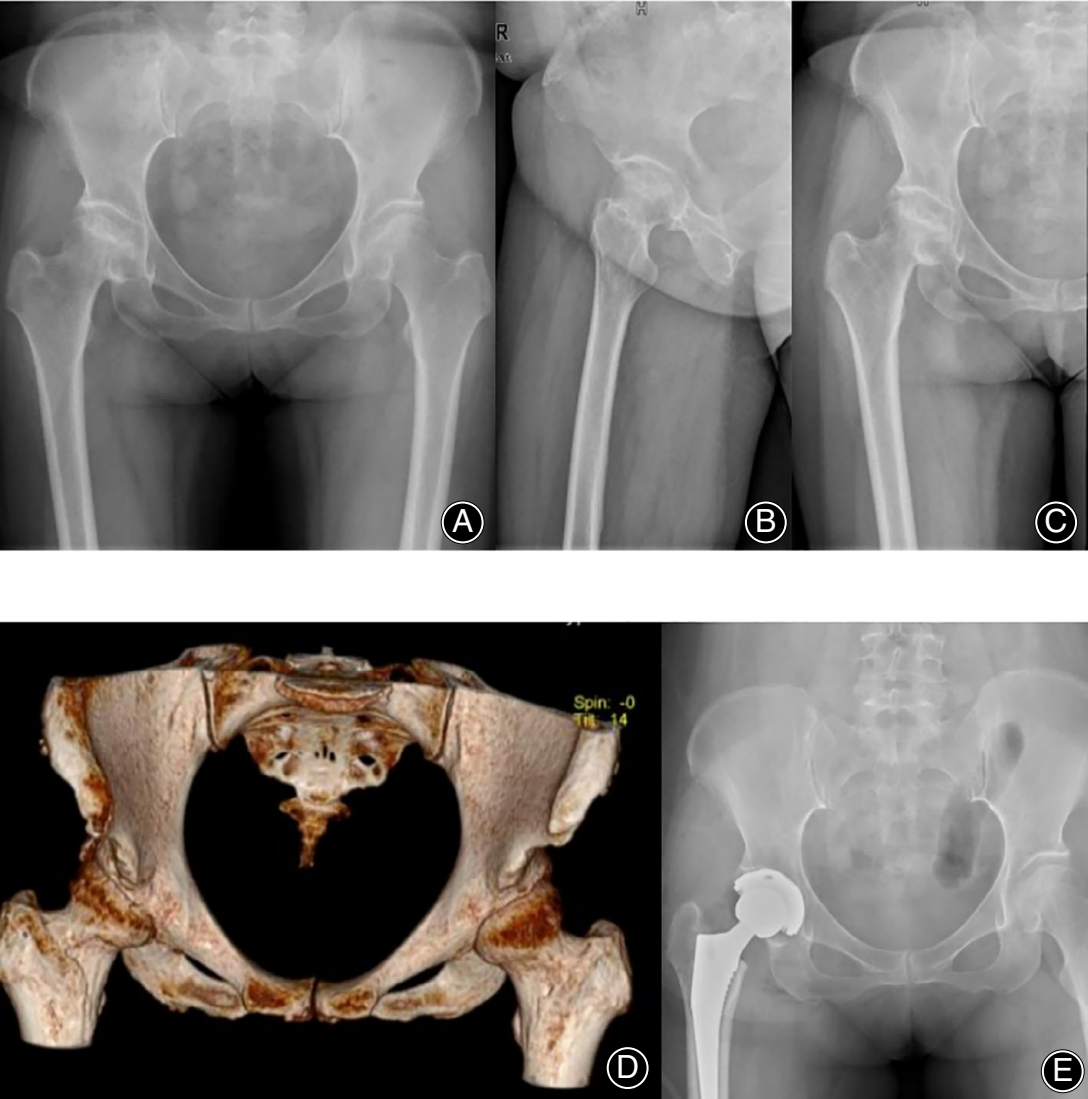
The images of hip PVNS after THA. (A–C) Preoperative pelvic radiograph depicting the right hip to PVNS. (D) Preoperative CT 3D reconstruction of hip PVNS. (E) Postoperative pelvic radiograph
